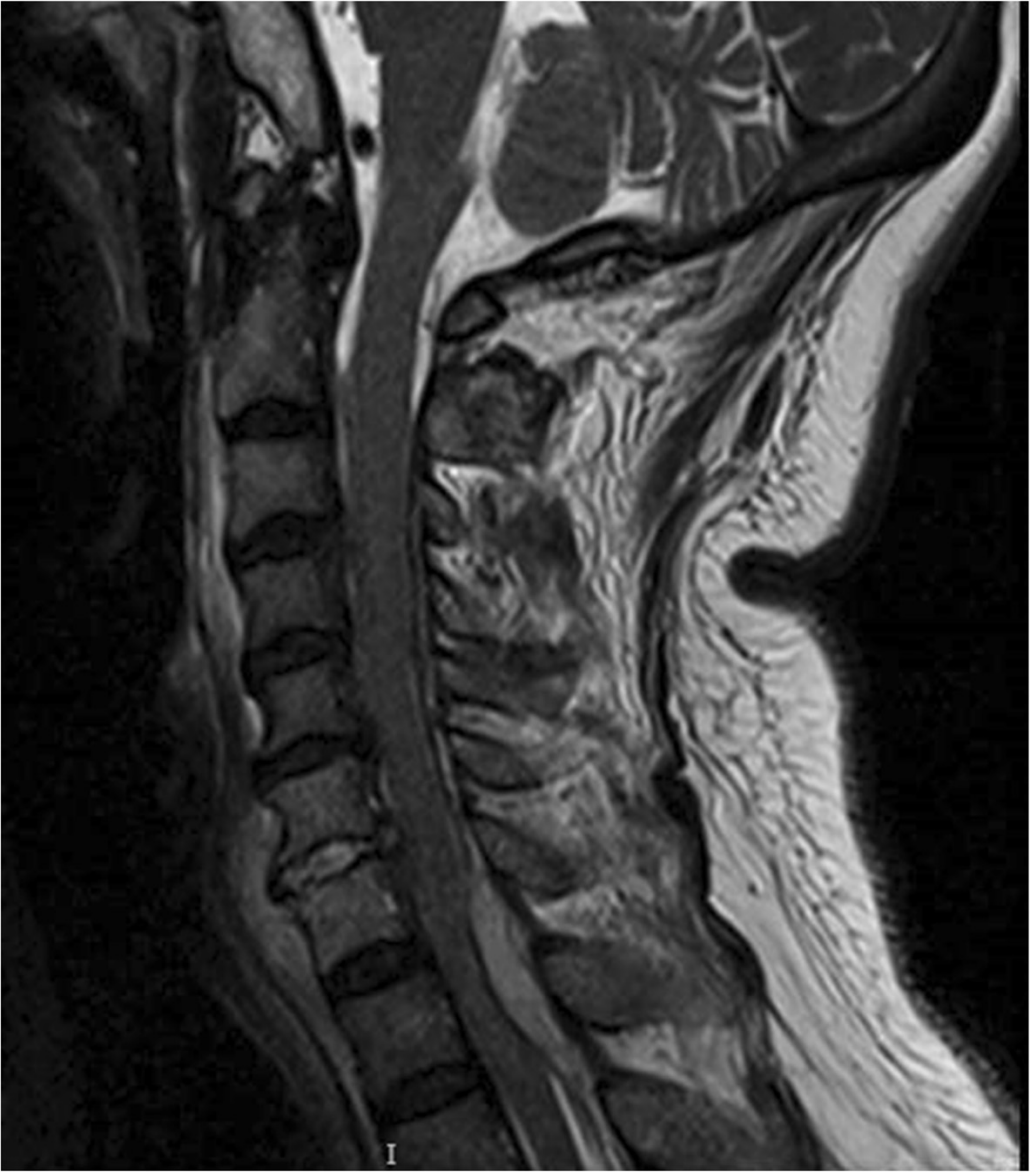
Sagittal view of the cervical spine on MRI, showing the epidural abscess which is compressing on the cervical cord anteriorly
